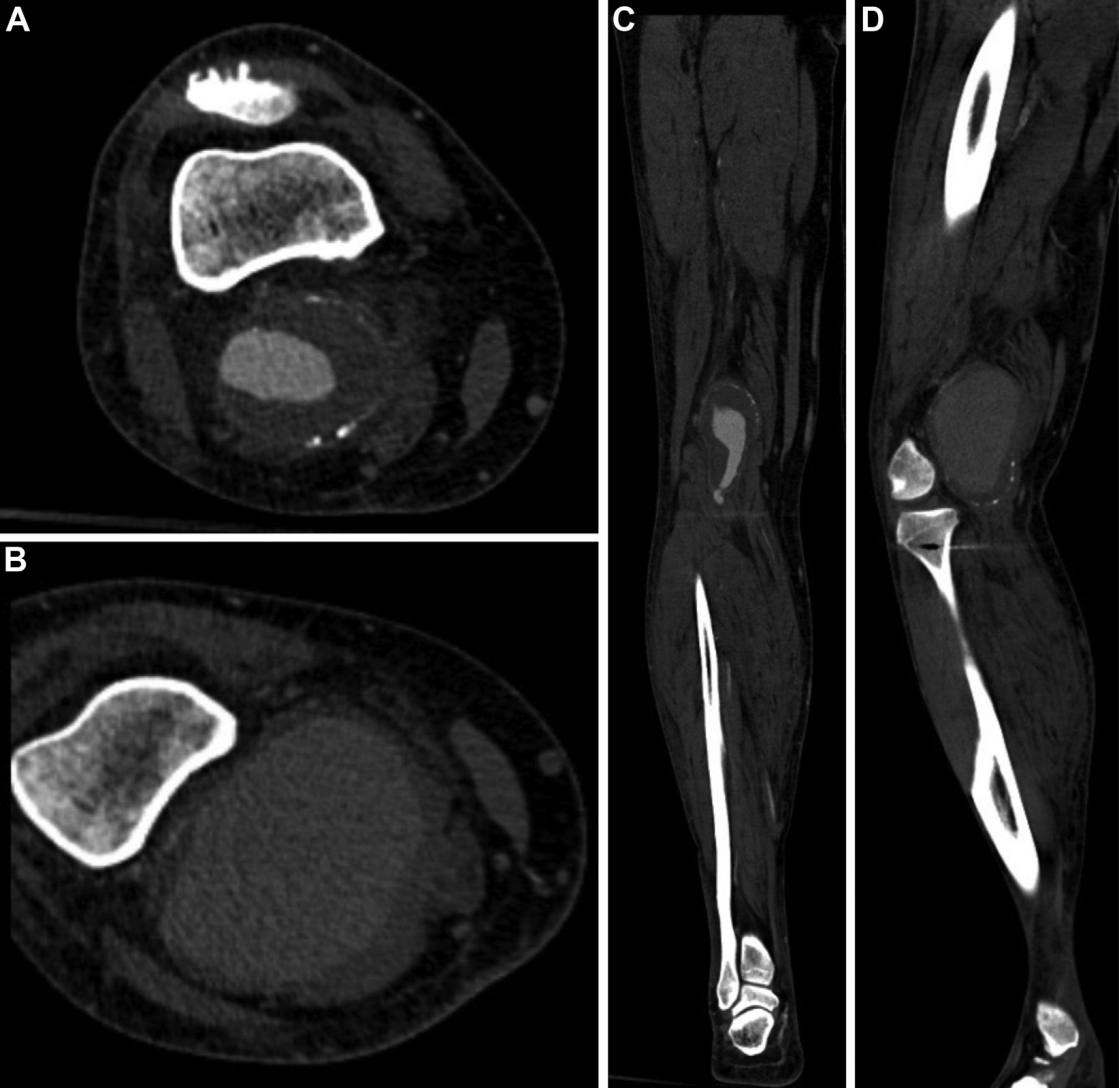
Computed tomography angiographic images of the right popliteal artery aneurysm (PAA) on axial (A and D) and coronal (B and C) projections demonstrating rapid expansion from 46 mm (A and B) to 81 mm (C and D) within a 2-week period. The aneurysm diameter was measured on the axial images perpendicular to the flow line to the largest diameter of the vessel with three-dimensional reconstructed computed tomography images.
